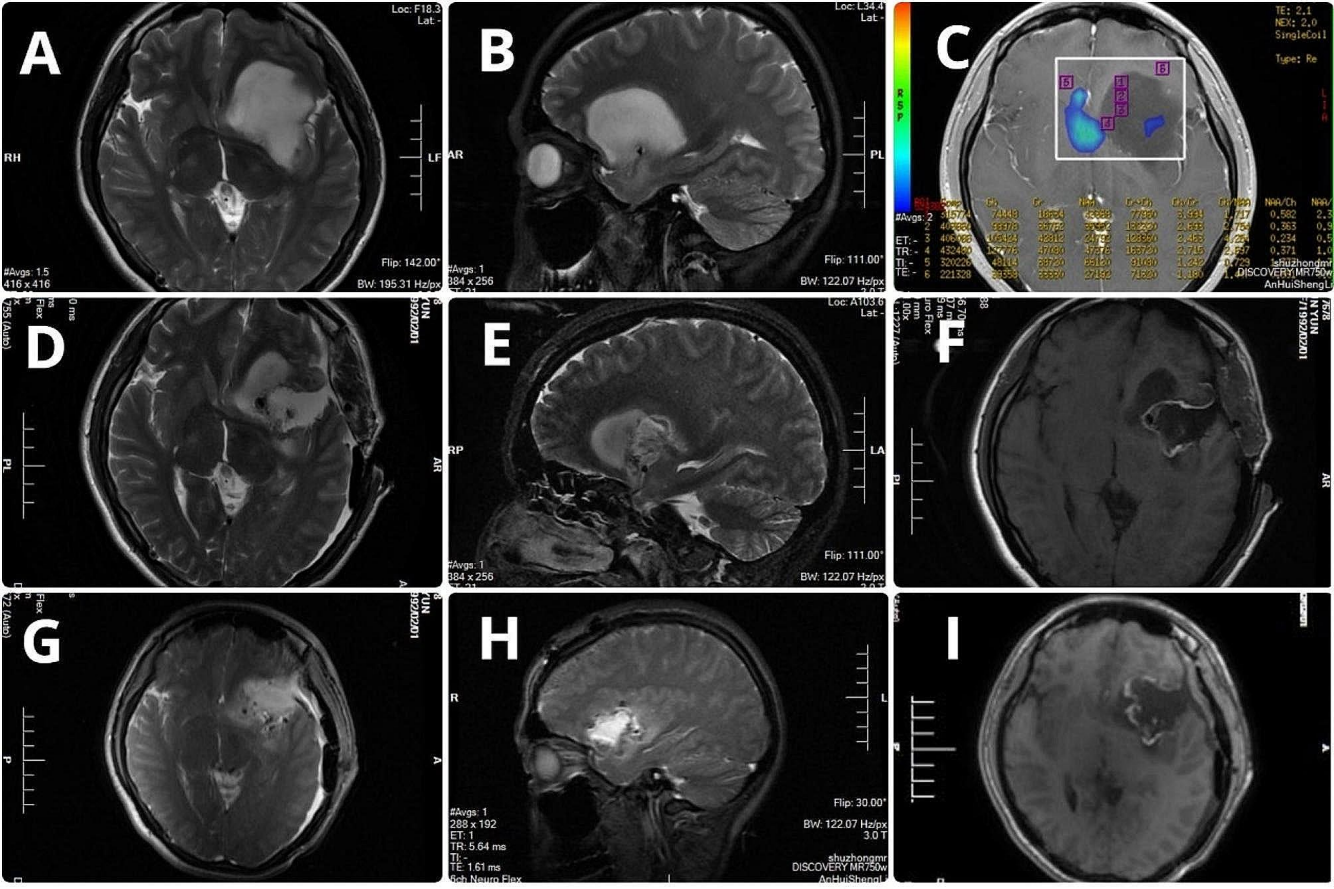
Case 2: Magnetic resonance imaging (MRI) of preoperative scan (A–C), first intraoperative MRI scan (D–F), and second intraoperative MRI scan. (G–I)
